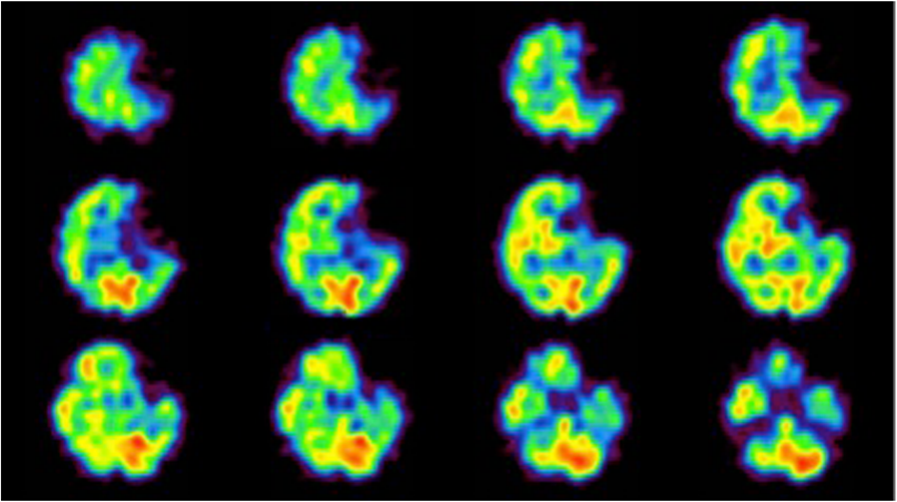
Postoperative single-photon emission computed tomography (SPECT) scan. The postoperative SPECT scan obtained from our hospital revealed that low cerebral blood flow lesions in the left cerebral hemisphere had not changed compared to the preoperative SPECT scan
